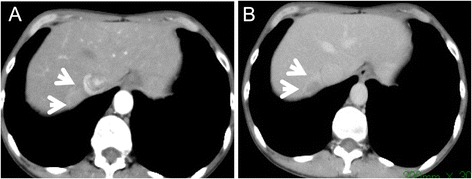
CT demonstrated hypodense nodule. Immediately enhanced in the early phase (A) and quickly de-enhanced in the late phase (B) after injection of contrast.
